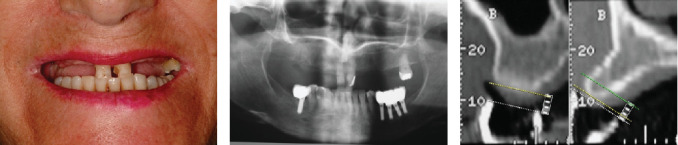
(a) Previous poor esthetic and functional condition. (b) Radiograph showing enough bone for implant placement. (c) Left, bone at 3 mm from margin; right, bone at 1 mm from margin.
